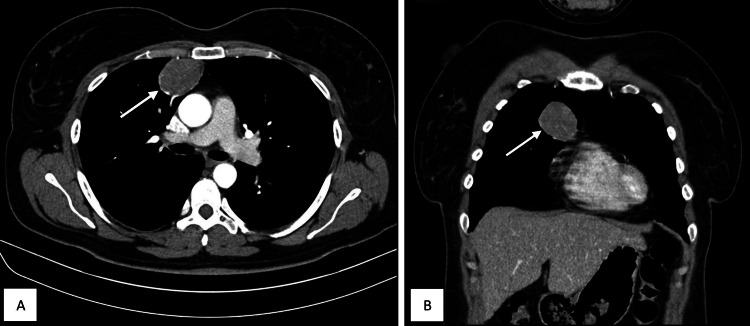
Axial (A) and coronal (B) CT scans showing a 5.5 x 5 x 2.5 cm mass (white arrow)
